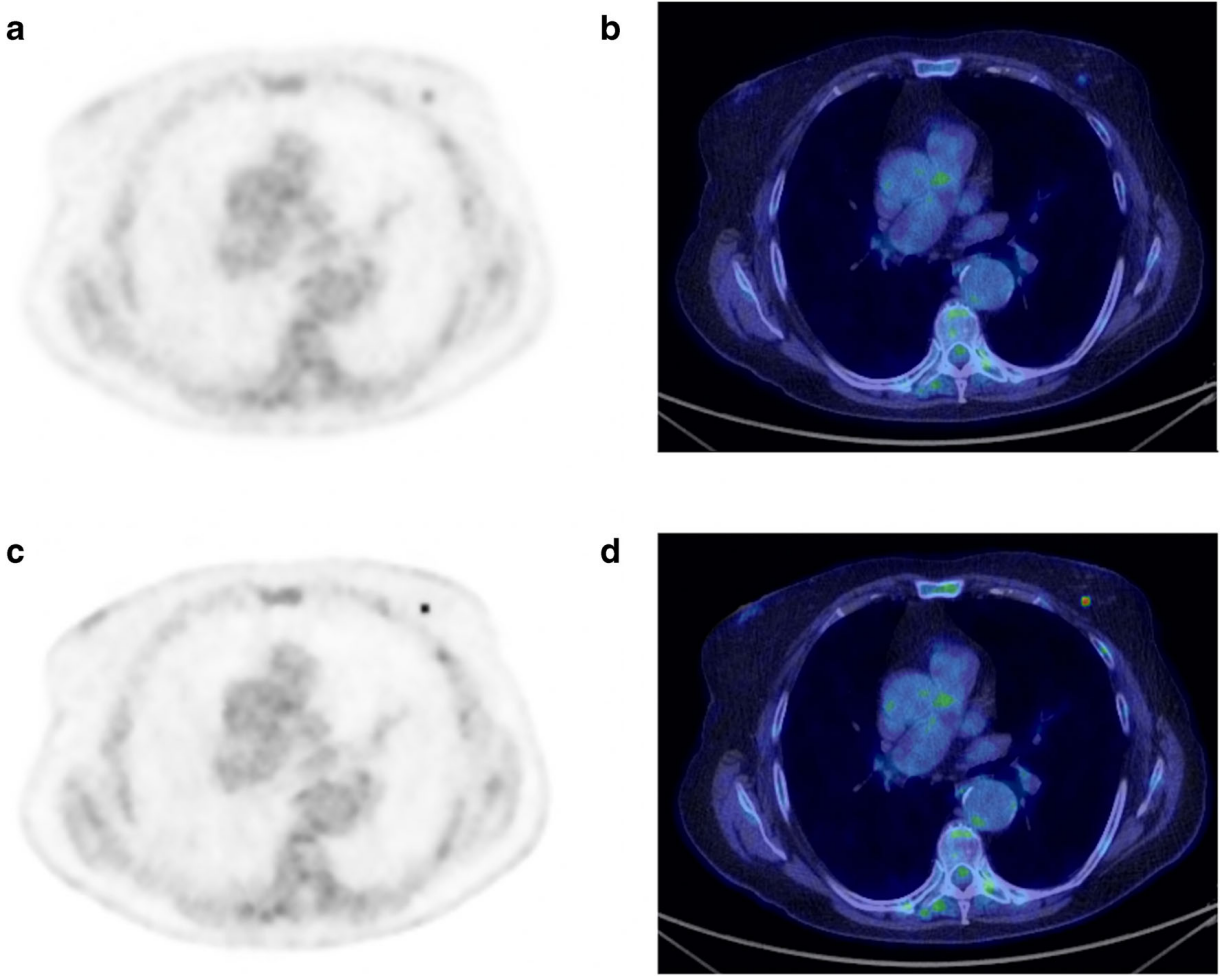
Incidentally detected tiny, ^18^F-FDG avid breast nodule in a 70-year-old patient. Triple assessment (mammogram, ultrasound and breast examination) following PET was negative, but 3 months later a small (sub-centimetre) ductal cell breast carcinoma was detected and cured. SUV_max_ 1.8 on OSEM (a and b) and SUV_max_ 5.0 on BPL (c and d). PET images on an SUV scale 0–6
